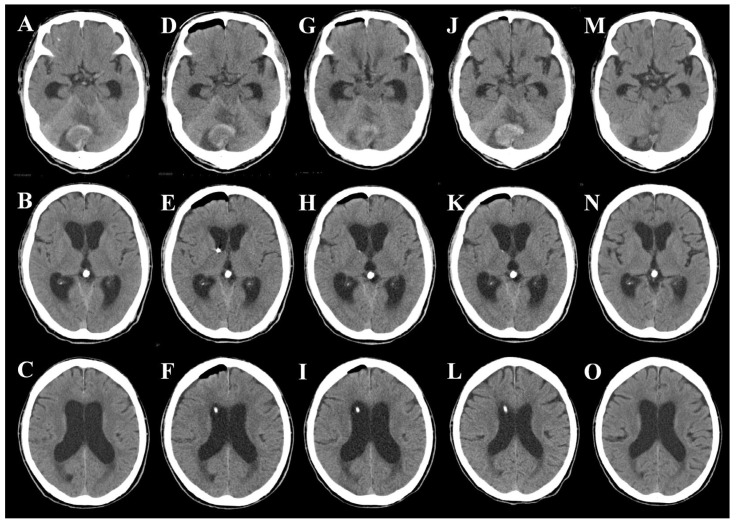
Serial computed tomography (CT) scans during hospitalization. (A–C) Day 1 (before EVD): CT showing right cerebellar infarction with fourth ventricular compression and acute obstructive hydrocephalus. (D–F) Day 1 (after EVD): CT confirming appropriate ventricular catheter placement with partial reduction in ventricular size. (G–I) Day 2: Persistent ventricular dilatation due to limited drainage, later improved following additional cerebrospinal fluid removal. (J–L) Day 6: CT after EVD removal showing maintained ventricular size without new hemorrhage. (M–O) Day 26: Follow-up CT demonstrating resolved hydrocephalus and reduction in mass effect in the posterior fossa.
